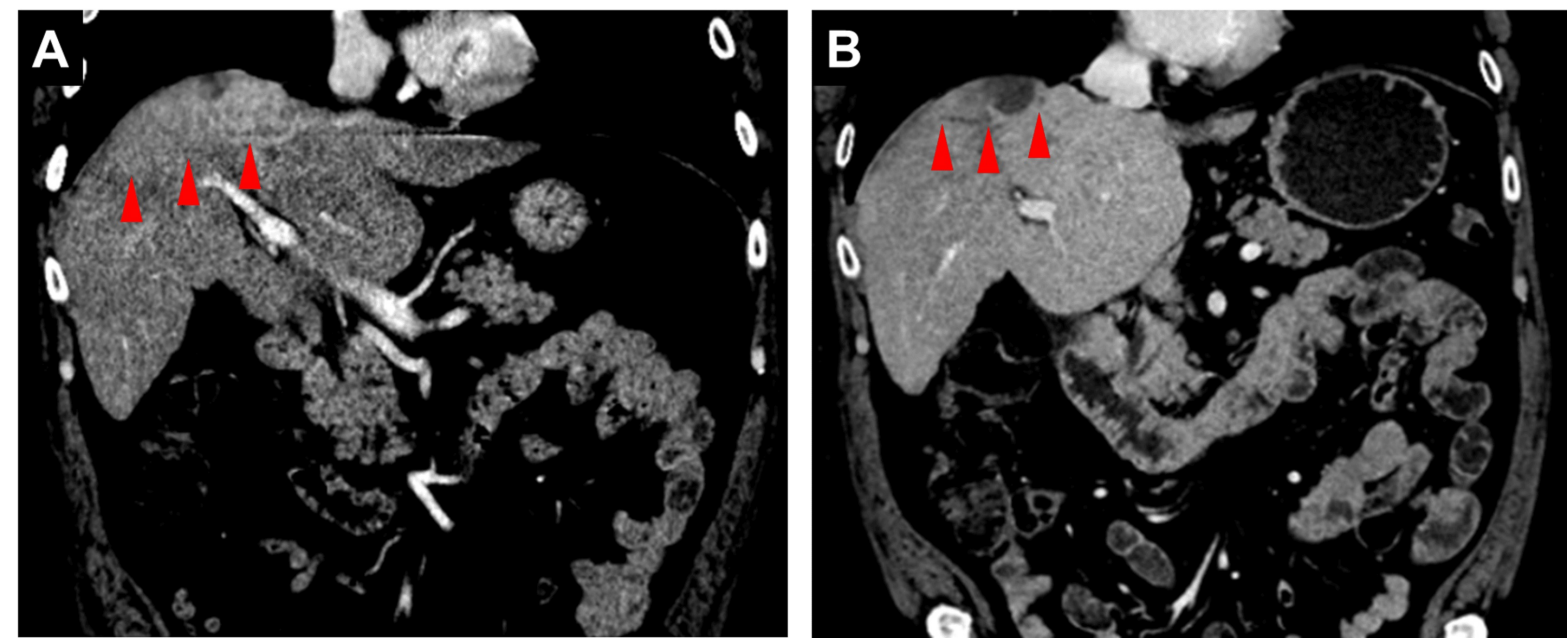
Restaging contrasted CT scans (A) Before treatment, the CT scan shows a multilobulated hepatic mass on the right lobe of the liver measuring 5.4×7.5×4 cm in size (red arrows). (B) After systemic therapy and radiation therapy, scans reduced the disease burden.
